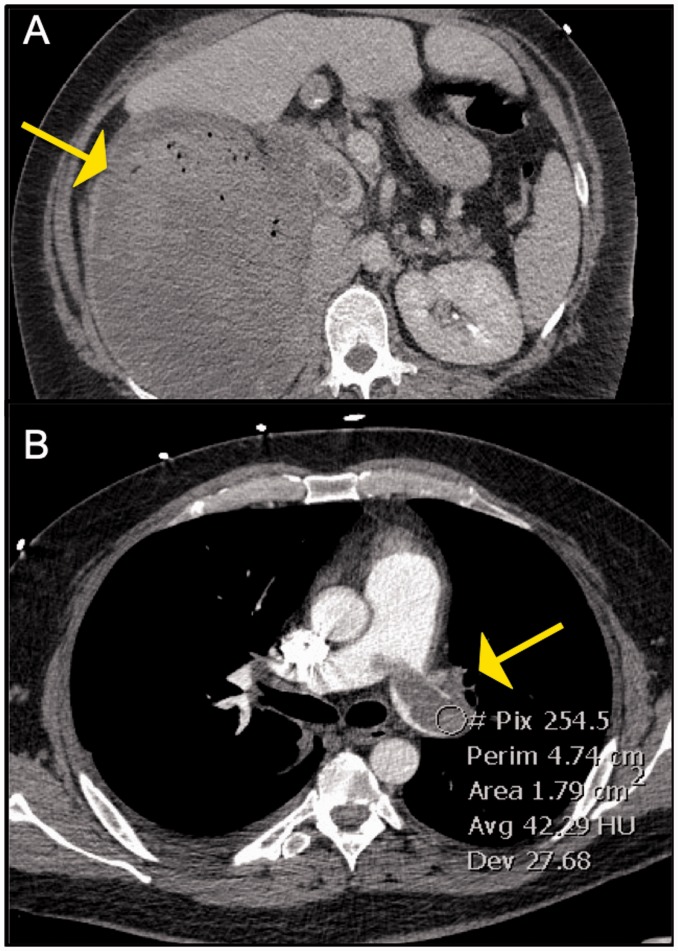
Panel A: Axial image from CT abdomen showing a large heterogenous right
